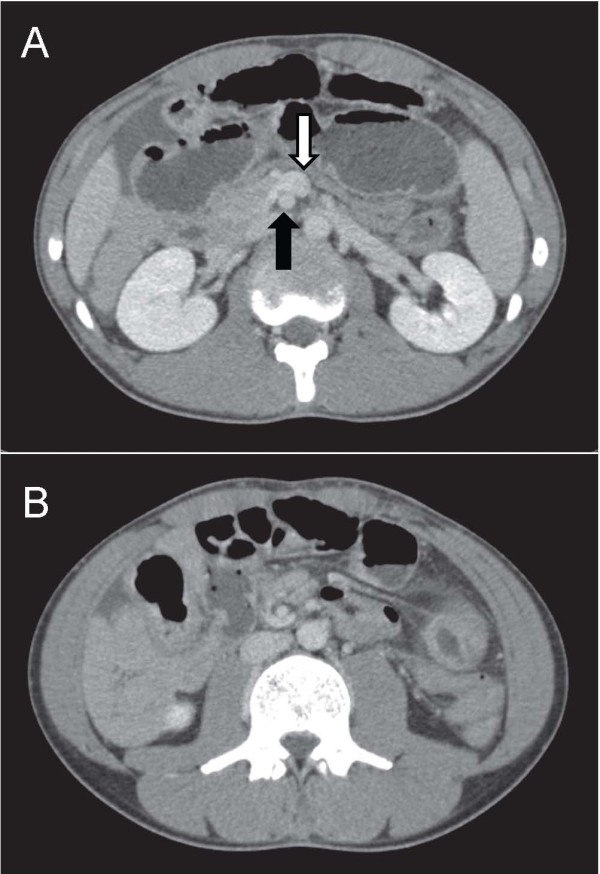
Contrast enhanced CT of the abdomen. A: Contrast enhanced CT can show the abnormal anatomic location of a right sided small bowel, a left-sided colon, and an abnormal relationship of the superior mesenteric vein (white arrow) situated to the anterior of the superior mesenteric artery (black arrow) instead of to the right. B: Opacified small bowel present almost entirely on the right side.
